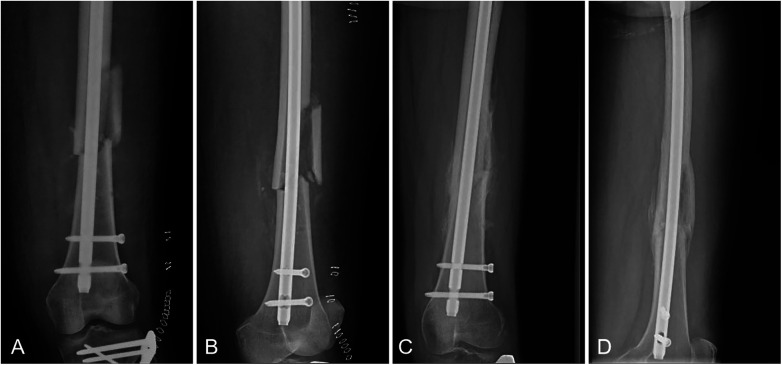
A case of fracture union.(A,B) Immediate postoperative anteroposterior (A) and lateral (B) radiographs of a patient who achieved fracture union. (C,D) Follow-up anteroposterior (C) and lateral (D) radiographs of the same patient at 10 months, demonstrating successful fracture union with robust bridging callus formation.
